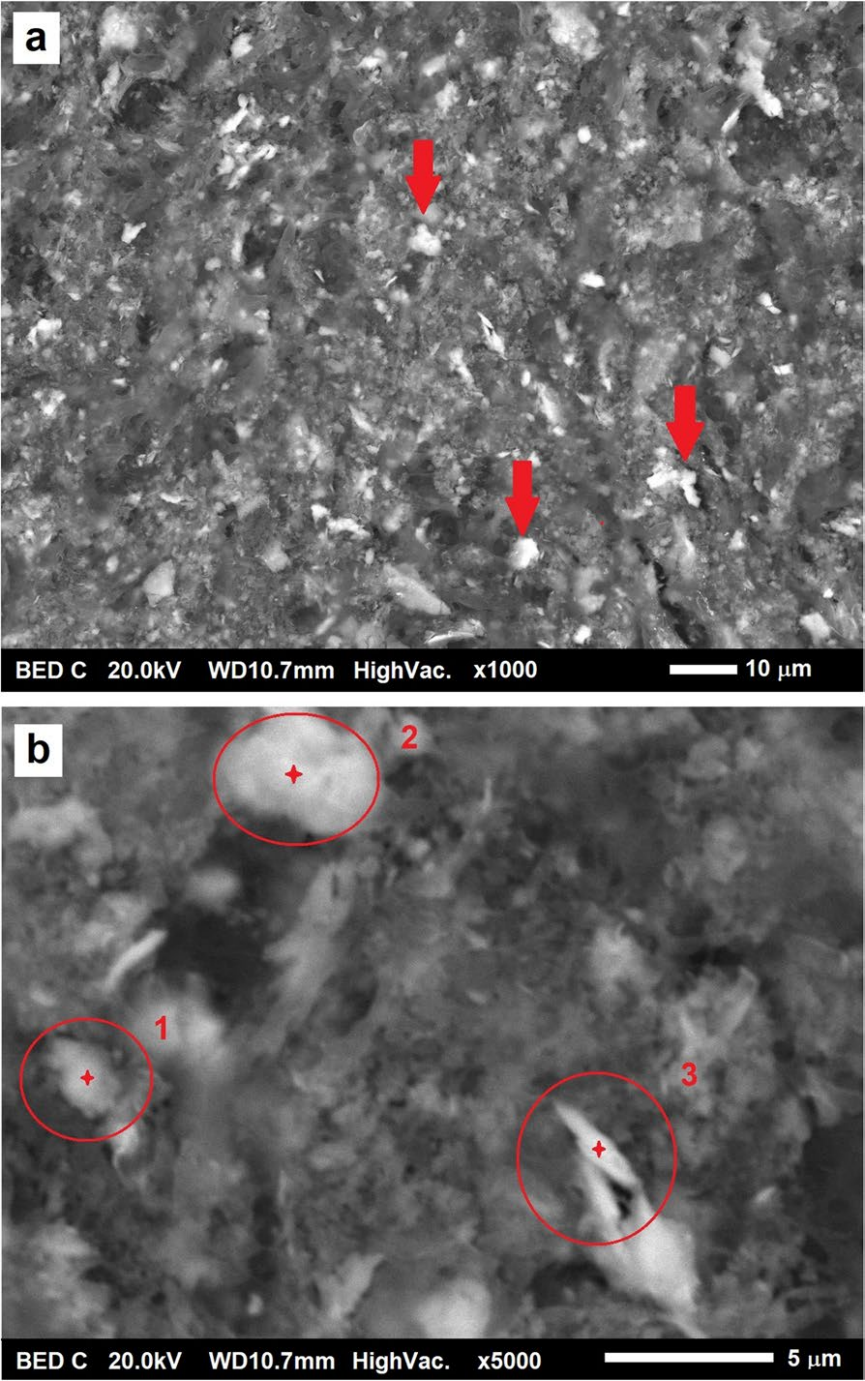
a Shows a BED image of the selected area of a KOH digested sample at medium magnification. The brighter particles, some of which indicated by red arrows, are metallic debris. b EDX acquisition spots marked in red. Spot 2 corresponds to the particle cluster providing a brighter contrast in the X-Ray Cr and Co maps (see the red circles of Fig. 4c and d)
